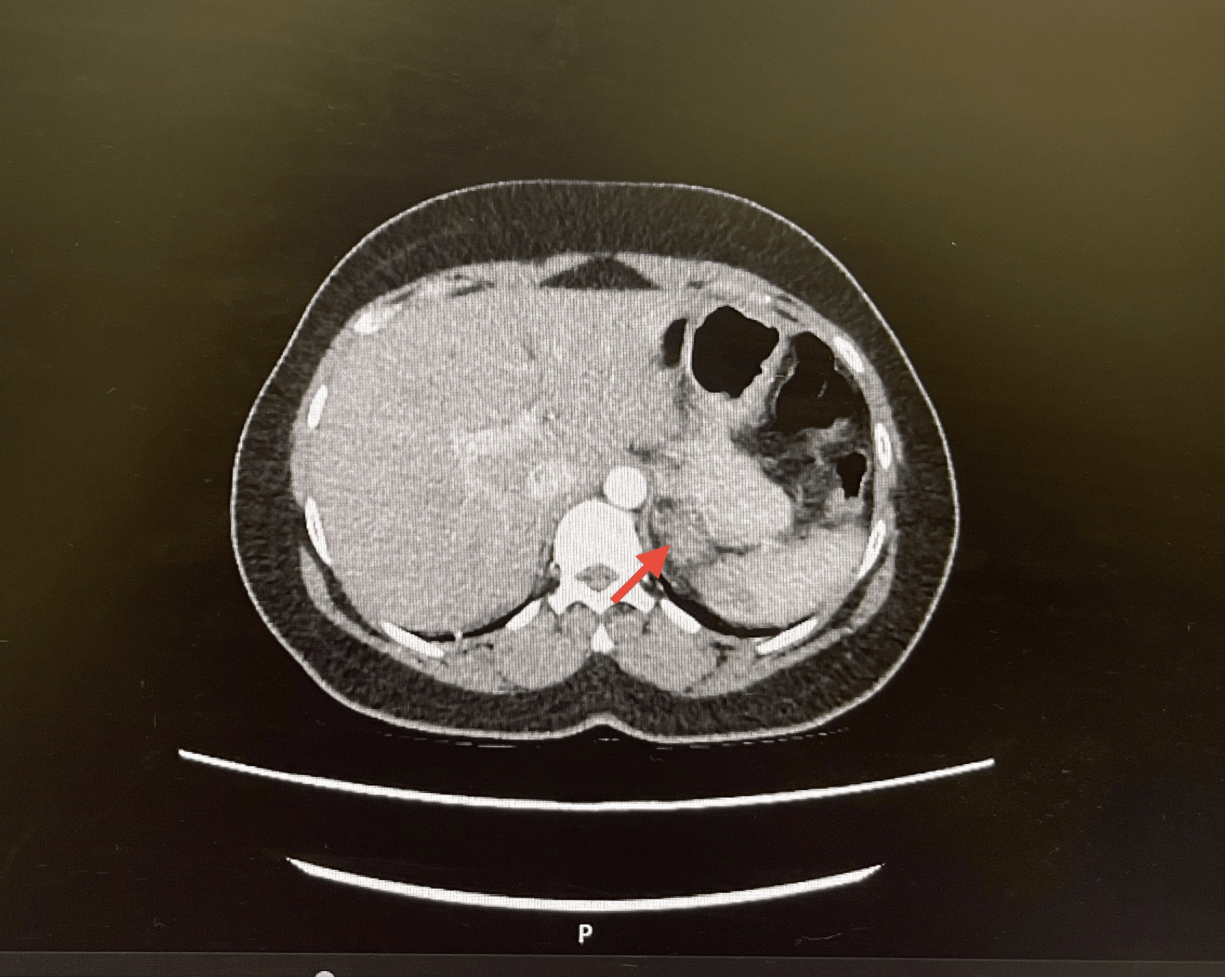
Contrast-enhanced CT scan showing left adrenal nodule (arrow), initially interpreted as a possible metastatic focus.
